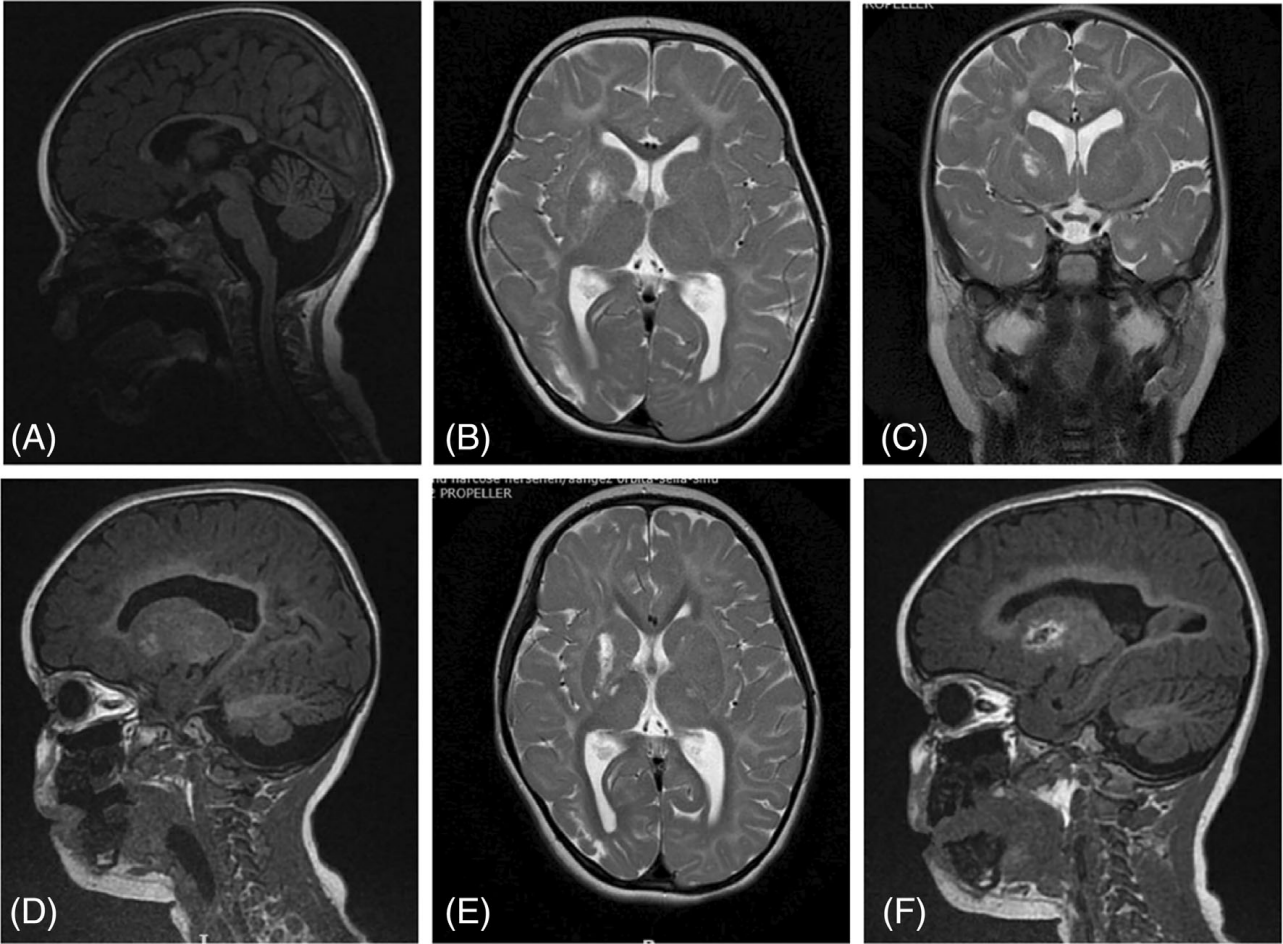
Sagittal T1 (A, D, F), axial T2 (B, E) and coronal T2 (C) images of Patient 1 at 2 years (A–C) and 4 years (D–F) of age. The initial MRI at 2 years (A–C) shows a thin corpus callosum and delayed myelination for the age – absent subcortical white matter myelination on T1‐weighted images and absent posterior limb of the internal capsule myelination on T2‐weighted images. Follow‐up MRI at the age of 4 years (D–F) demonstrates no significant increase of volume of cerebellum and vermis, no significant progress in myelination. Increased T1 weighted signal abnormalities in basal ganglia and thalamus.
